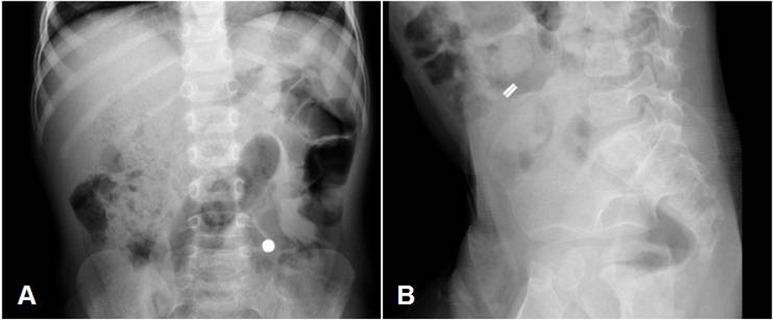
Figure 1: A.Abdominal X-ray showing a disc-shaped foreign body in the left lower quadrant. B.Lateral abdominal X-ray in which a separating line was noted inside the foreign body.
